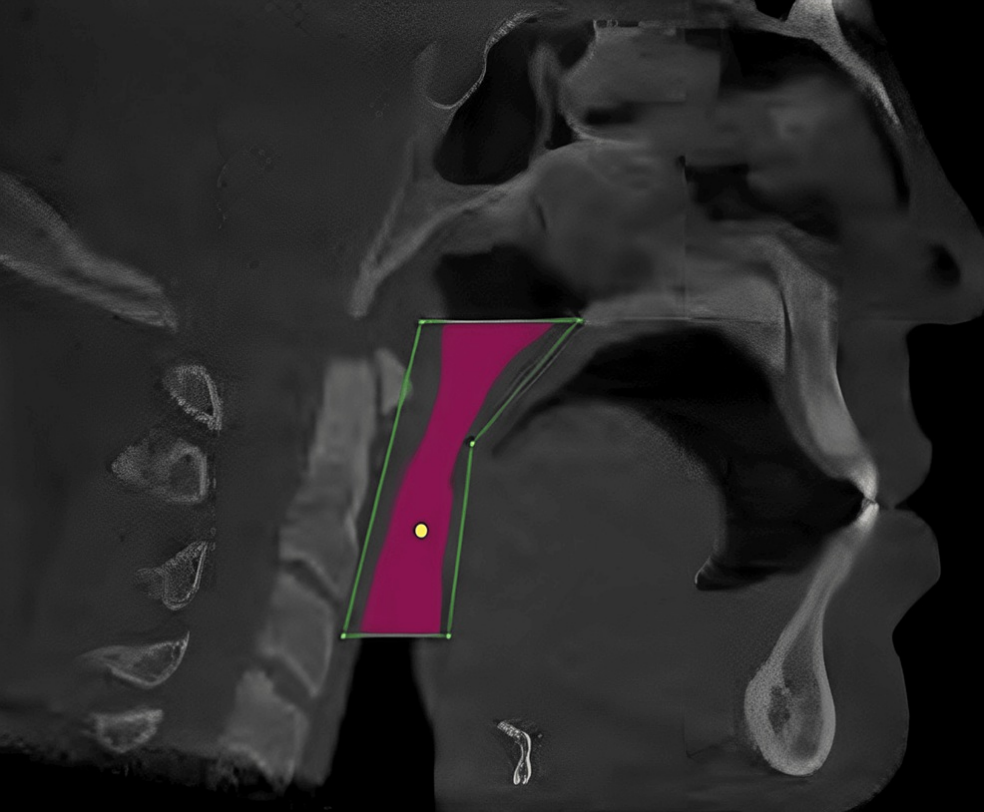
Image of the cone-beam CT depicting the pharyngeal space
